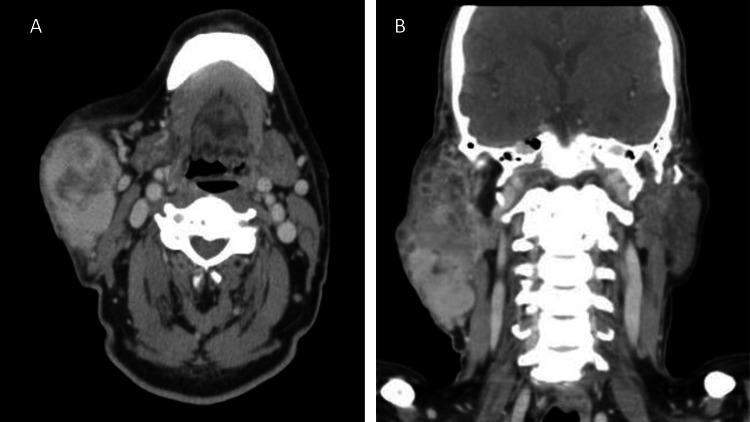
CT scan in axial and coronal views Axial view shows a heterogeneously enhancing and solid cystic lesion (A). Coronal view shows multiple, solid enhancing nodules adjacent to the main lesion (B).
